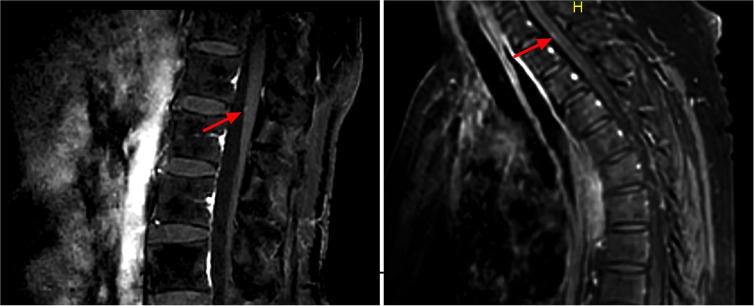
Cervical spine MRI of the patient. Nov 2023: Diagnosis of B‑cell ALL; induction chemotherapy (VDP regimen) + high‑dose methotrexate (3g/m²) + 7 cycles of intrathecal chemotherapy (MTX 15mg, Ara‑C 40mg, DEX 5mg). May 2024: Complete bone marrow remission achieved; consolidation chemotherapy (4 cycles of high‑dose cytarabine); maintenance chemotherapy initiated. Aug 2025: Isolated CNS relapse (neck pain, vomiting, dizziness; CSF flow cytometry: 74% immature lymphocytes; normal bone marrow); 7 cycles of twice‑weekly intrathecal chemotherapy Late Sep 2025: 1 month after last intrathecal injection; onset of progressive myelopathy (bilateral lower limb weakness, T4 sensory level, urinary retention) Sep 2025: Cervical spine MRI: C7-T2 dorsal column T2 hyperintensity, no enhancement. Dec 2025: 3‑month follow‑up; persistent lower limb weakness (MRC grade 3/5), partial improvement of urinary retention
